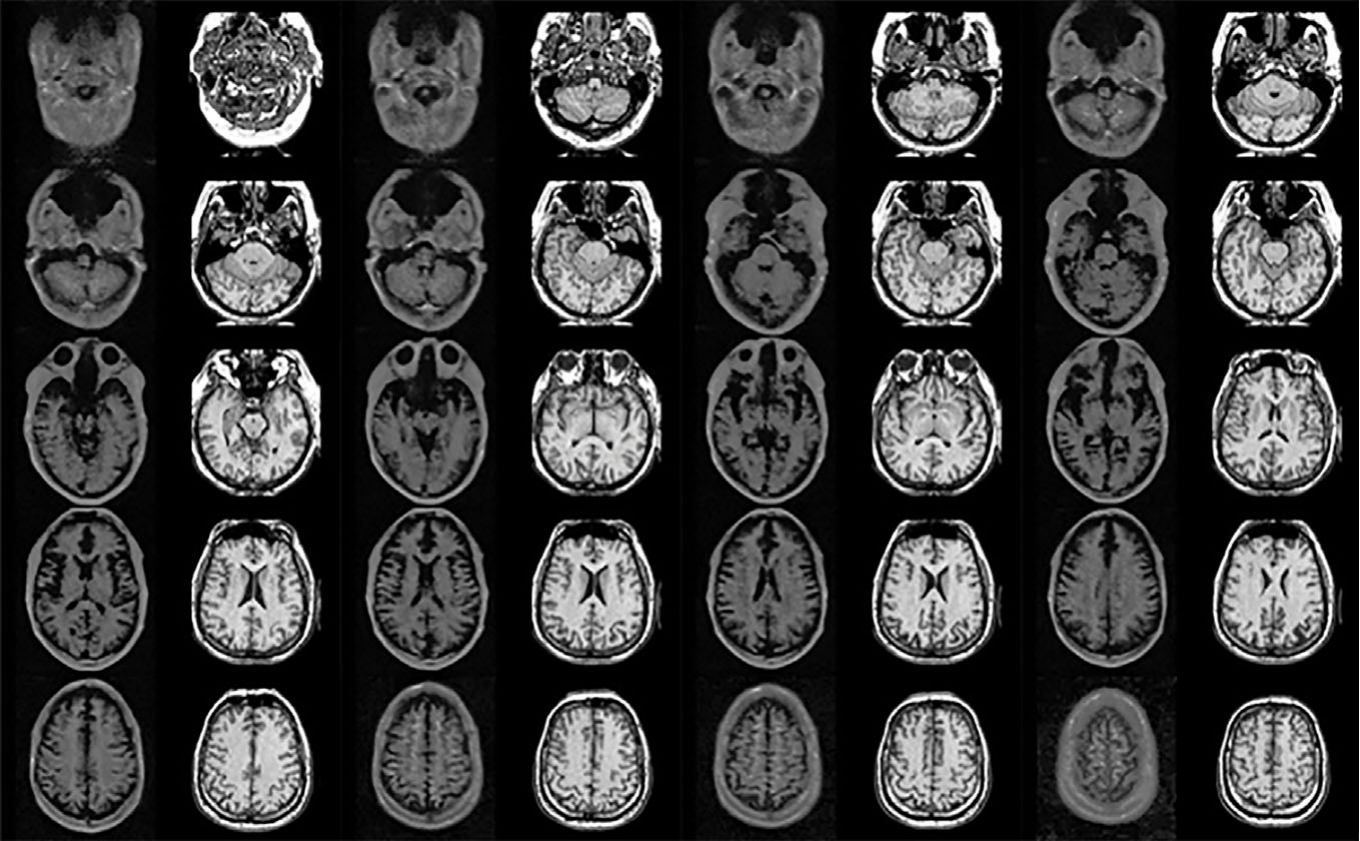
Unpaired image set used for training (left and right sides show 0.06 Tesla and 1.5 Tesla MR images, respectively).
